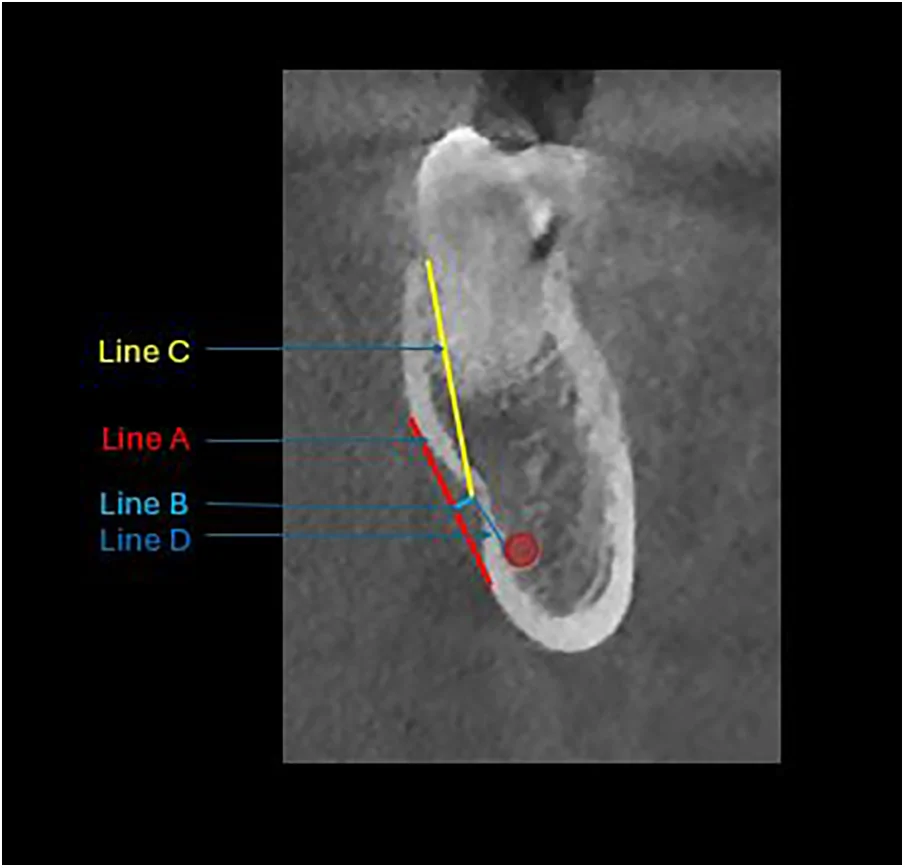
Submandibular fossa, distance from alveolar crest and relationship between mandibular canal and submandibular fossa. Line A (yellow): Reference line connecting superior and inferior points of lingual concavity. Line B (red): Submandibular fossa (SF) depth (perpendicular distance from deepest point to Line A; shown as 3.0 mm). Line C (blue): Alveolar crest (AC)-to-fossa distance (shown as 17.2 mm). Line D (green): Mandibular canal (MC)-to-fossa distance (shortest distance from most lingual canal point to deepest fossa point; shown as 1.3 mm). SF, submandibular fossa; MC, mandibular canal (inferior alveolar nerve canal); AC, alveolar crest; CBCT, cone beam computed tomography. Measurements in millimeters using I-CAT Vision software.
